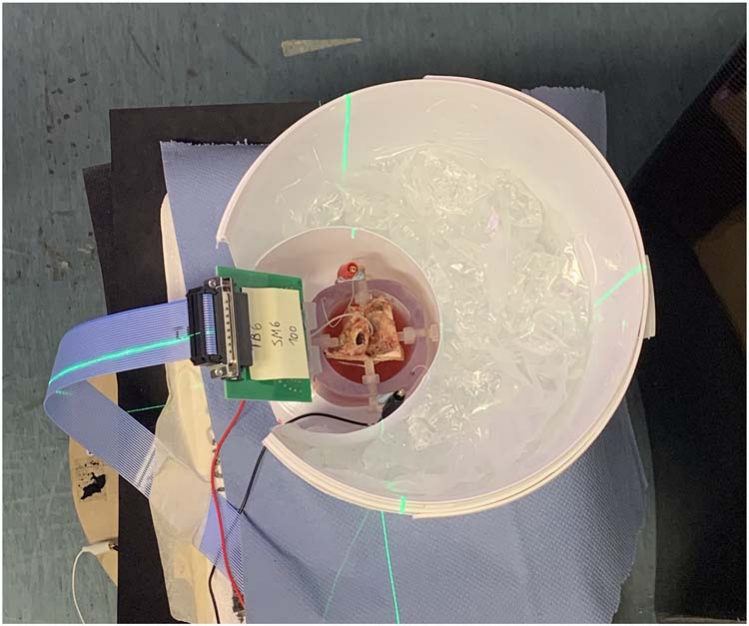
A temporal bone in Ringer’s solution bath enclosed by a water-sac placed in the prototype XFi (Planmeca Oy, Helsinki, Finland) CBCT device.
